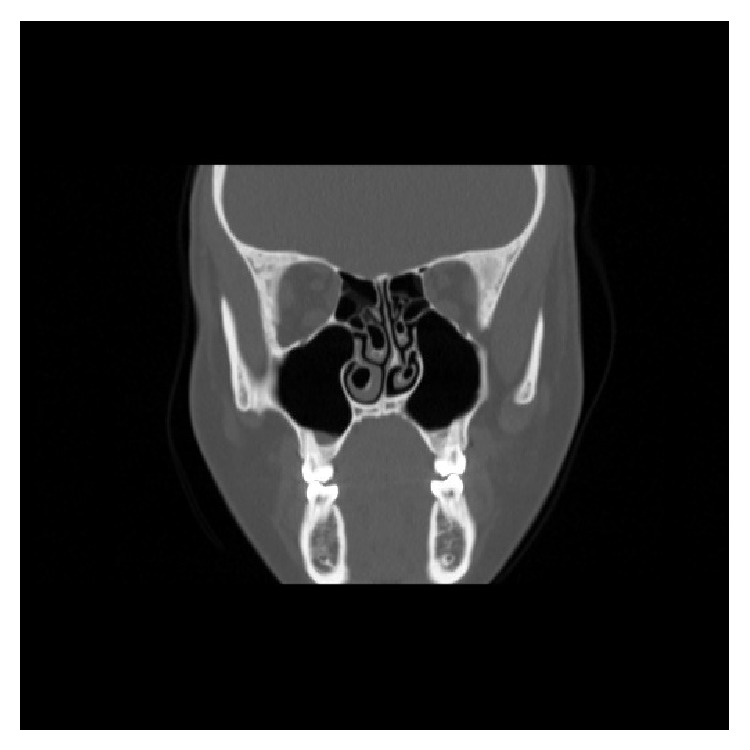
Coronal computed tomography section showing bilateral middle and inferior turbinate pneumatization and nasal septal deviation to left.
